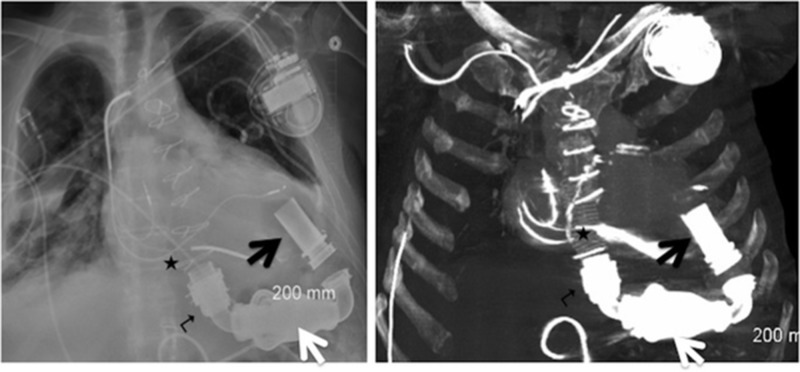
Plain radiograph and maximum intensity projection (MIP) computed tomography (CT) images showing Heartmate II left ventricular assist device (LVAD). Black arrow – inflow cannula; white arrow – pump; turn arrow – band relief; black star – outflow cannula.
